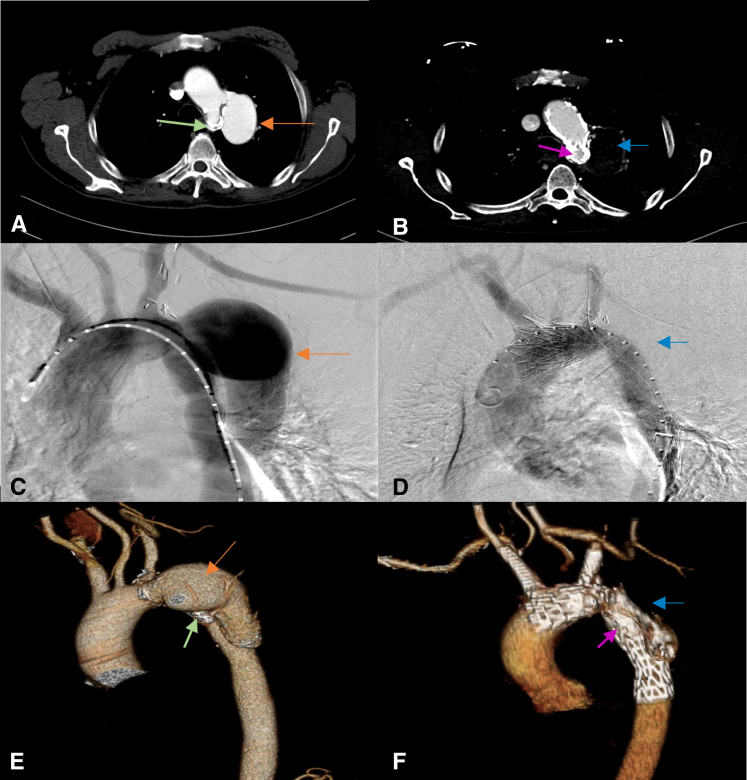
Preoperative computed tomography (*CT*) (A), digital subtraction angiography (*DSA*) (C), and 3-dimensional reconstruction (E) demonstrate pseudoaneurysm of the extra-anatomic bypass (*orange arrow*) implanted to address anastomotic coarctation of in situ graft (*green arrow*). Postoperative CT (B), DSA (D), and 3-dimensional reconstruction (F) demonstrate successful stent dilation of recoiled interposition graft (*purple arrow*) and exclusion of the extra-anatomic bypass graft (*blue arrow*) using a thoracic branched endoprosthesis device.
